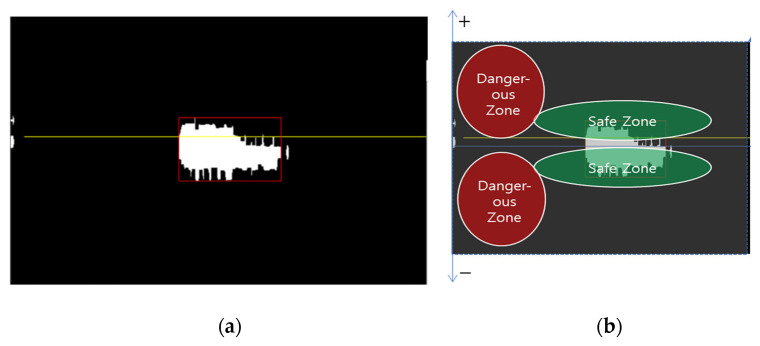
Concept of flood risk determination using WAVE-Surf: (a) example of detected pure flow energy; (b) flood risk on the range–Doppler map.
